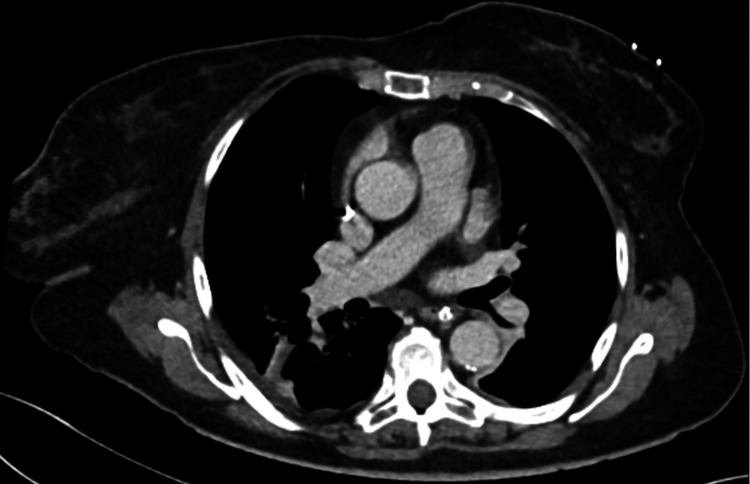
Chest CT angiogram in previous hospital
